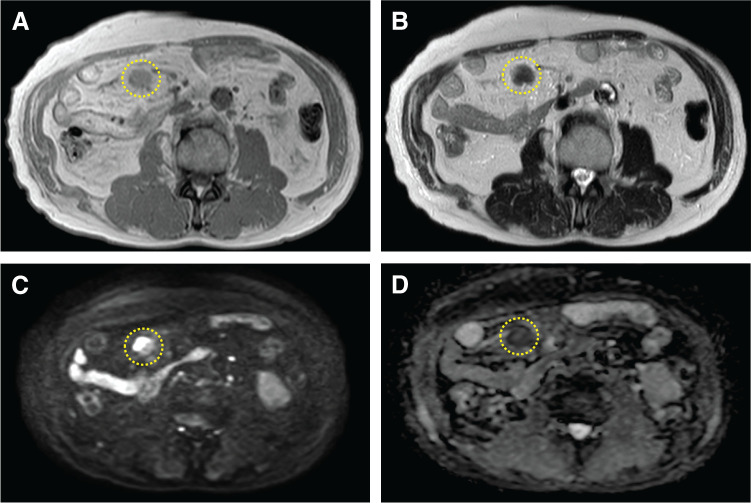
CE-MRI images show a mesenteric mass (yellow circle outlines) with slightly higher signal intensity than muscle on T1WI (A) and with an isointense signal on T2WI (B). The lesion shows diffusion restriction on DWI (C) and reduced ADC values on the ADC map (D). ADC, apparent diffusion coefficient; CE-MRI, contrast-enhanced-MRI; DWI, diffusion-weighted imaging; T1WI, T1-weighted images; T2WI, T1-weighted images
